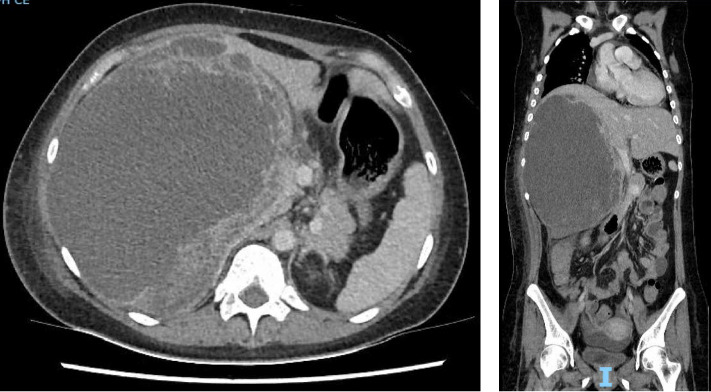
A very large cystic lesion in the right lobe of the liver measuring 15.2 × 17.3 × 21.7 cm with rim enhancement and multiple peripheral septations is seen on contrast-enhanced CT scan of the abdomen. (a) Transverse plane. (b) Coronal plane.
